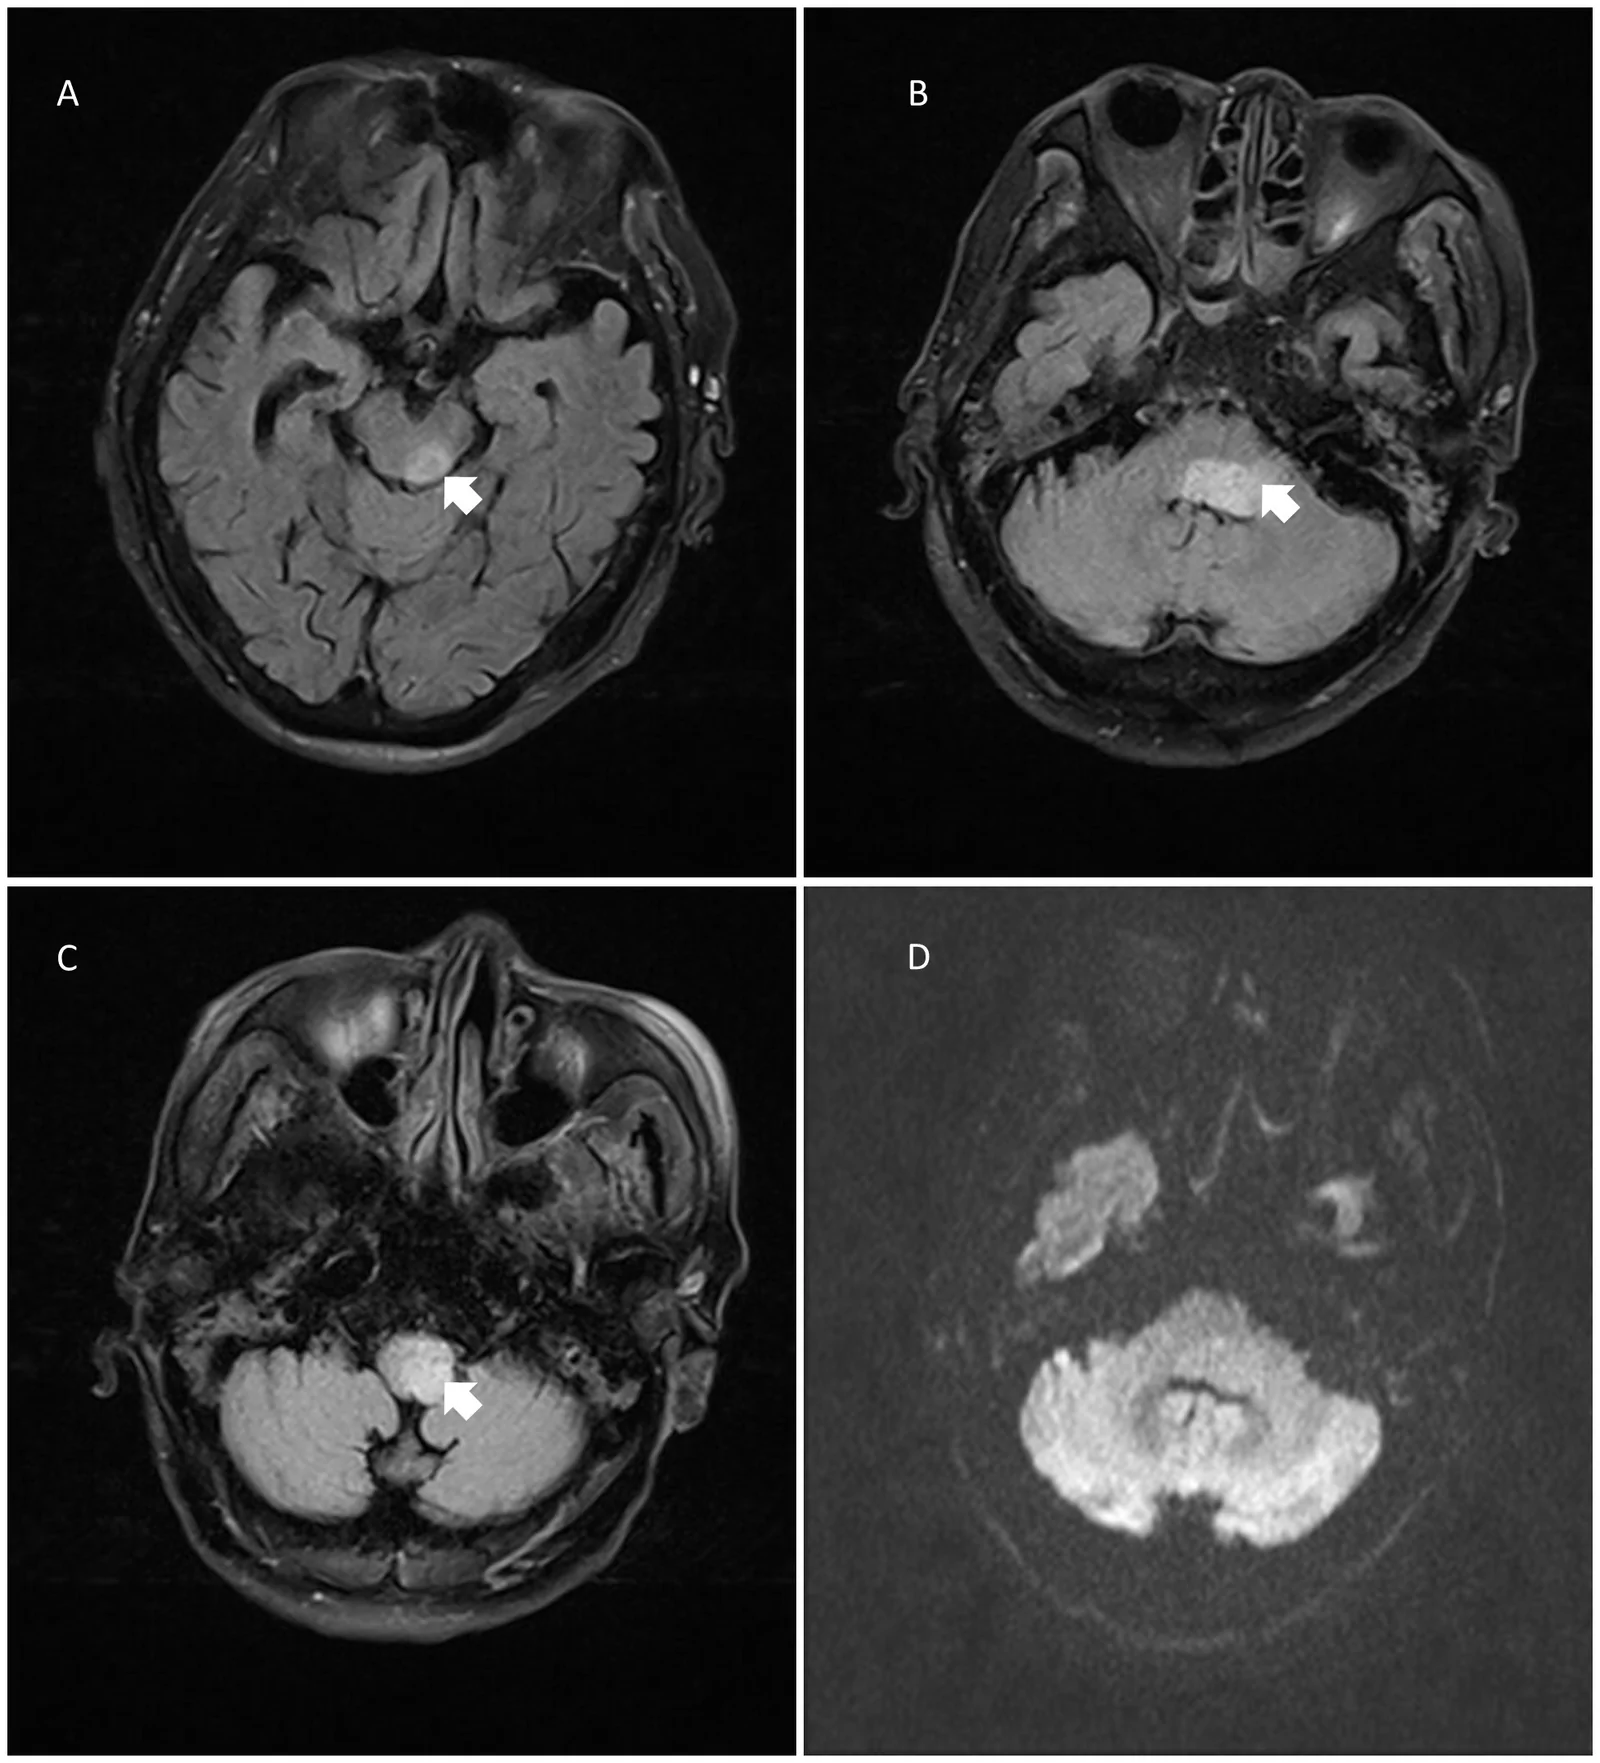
MRI of the brain showed brainstem involvement, including the left midbrain (A); arrowhead), pons (B); arrowhead), and the majority of the medulla oblongata (C); arrowhead), with hyperintensity on FLAIR. DWI (D) showed no evidence of diffusion restriction.
